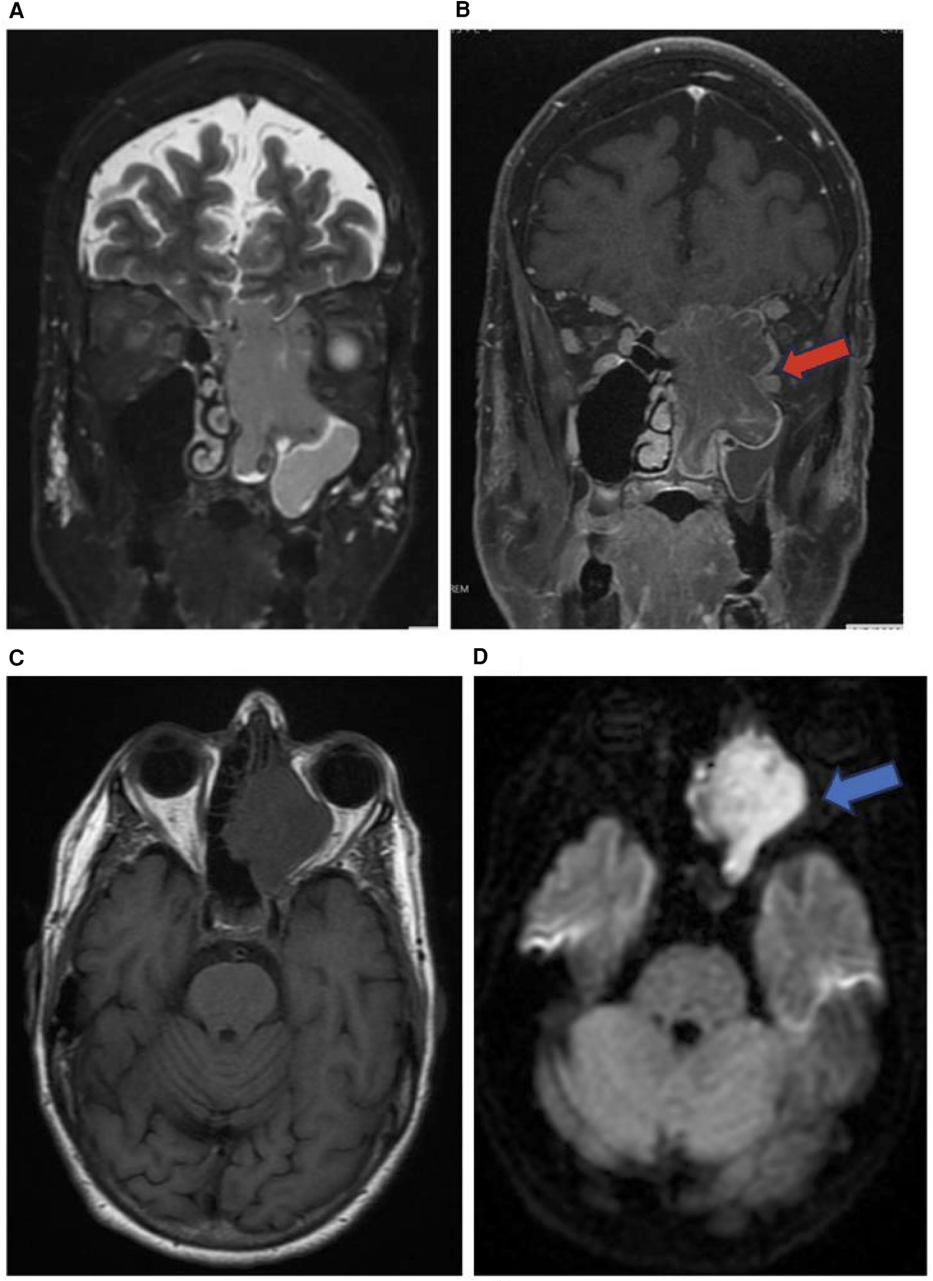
Sinonasal undifferentiated carcinoma. Coronal T2 (A), coronal T1 + C (B), axial T1 (C), & axial DWI (D) images showing a mass extending from the left maxillary sinus (red arrow) into the orbit (blue arrow) and intracranial fossa.
